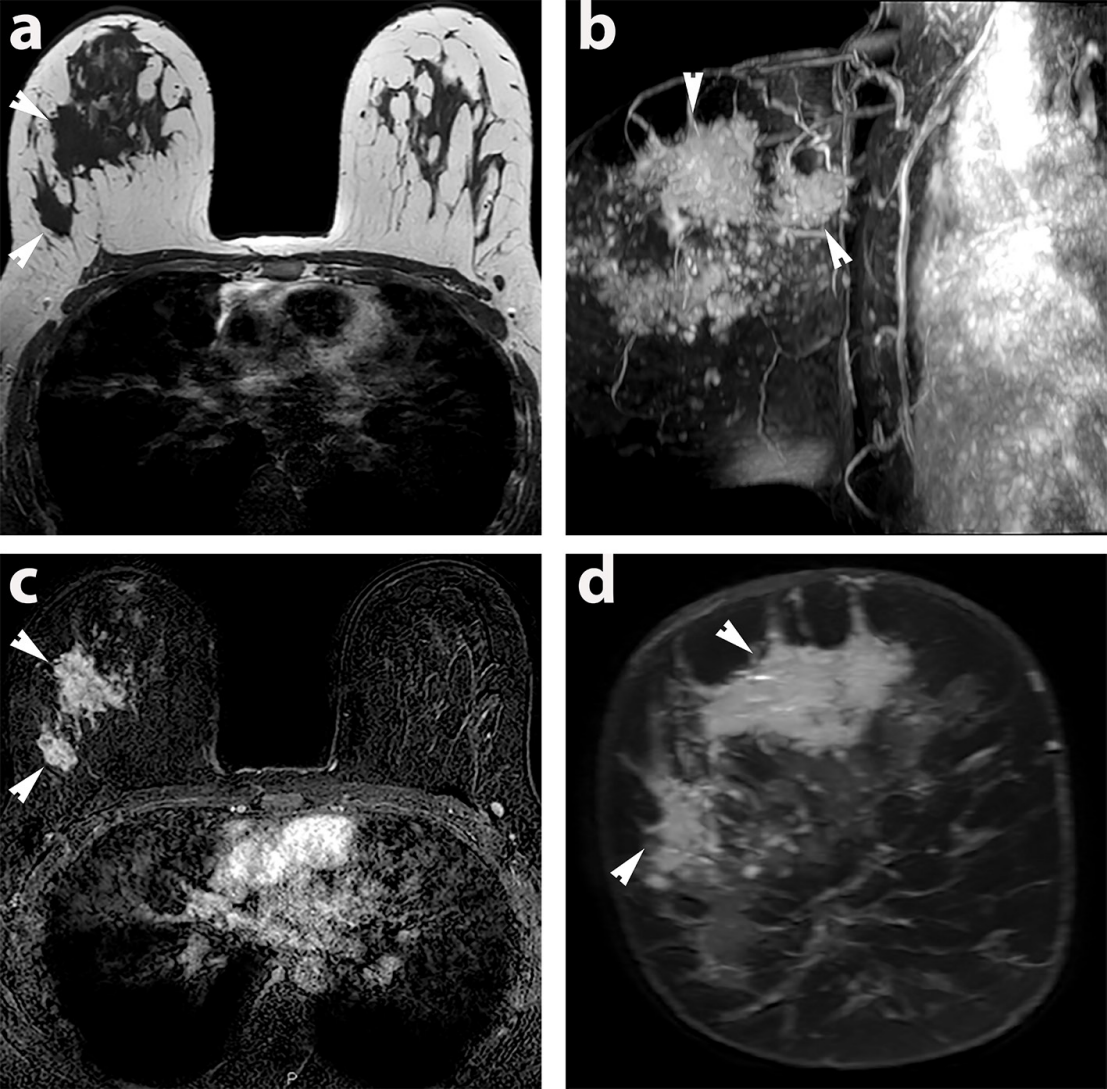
MRI of extensive multicentric right-sided breast cancer in the prone position (a) *T_1_* weighted axial image of upper part of breast (b) 3D MIP sagittal image of the 2 mins contrast enhanced images showing the extent of malignant disease in the breast. (c) 2 min contrast enhanced T1 subtracted axial image of the upper part of the breast (d) T1 post contrast reconstructed coronal image of the tumour. The regions of tumour imaged with HP MRI are highlighted with white arrowheads.
